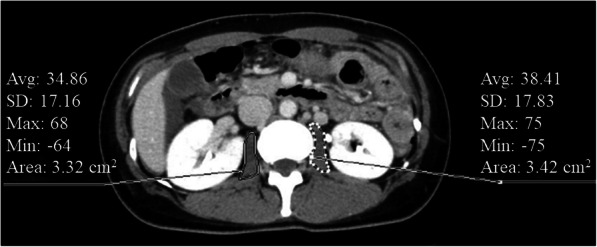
PMI measurement. Body muscle mass measured using axial CT imaging at the level of the third lumbar vertebra (L3)
